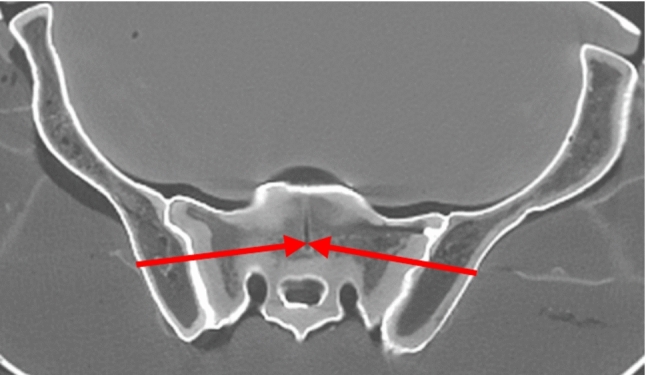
Axial CT image of the pelvis. Example for the S1 drilling corridor
